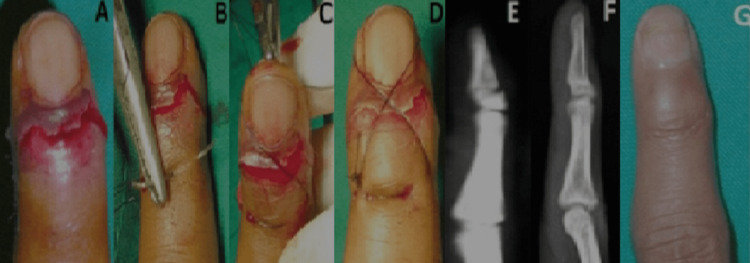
Case of a 21-year-old male with fingertip injury associated with nail bed avulsion (4A-4G) On examination, tenderness was present over the fingertip. On X-ray, a distal phalanx base fracture was observed. The patient was given a vertical figure-of-eight suture securing the nail plate with the nail bed after reducing the distal phalanx fracture. After four weeks, sutures were removed, and an X-ray was taken on follow-up. After six weeks, finger pinch movement was achieved.
